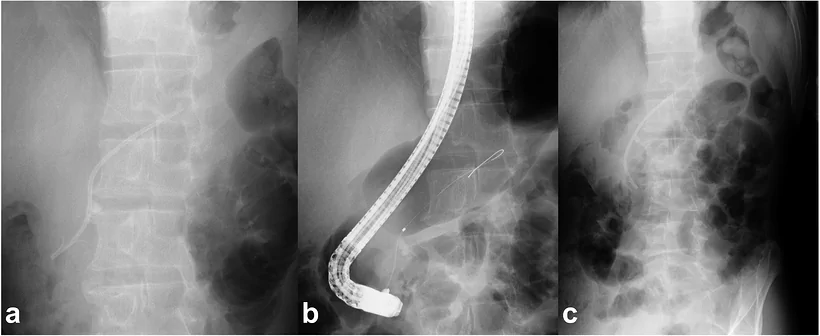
Radiographic and fluoroscopic findings during exchange of a pancreatic duct stent retained for 13 years. (a) Radiographic imaging showing the long‐term indwelling pancreatic duct stent that had been retained for 13 years. A pancreatic stone is shown adjacent to the retained stent. (b) A guidewire was inserted into the pancreatic duct, and endoscopic removal of the retained pancreatic duct stent was performed. No firm adhesion between the stent and the pancreatic stone was observed, and there was no evidence of stent fragmentation due to material deterioration during removal. (c) After removal of the retained stent, a new 7‐Fr 10‐cm pancreatic plastic stent was placed across the pancreatic duct stricture. Although stent insertion was technically challenging because of the pancreatic stone and ductal narrowing, successful stent placement was achieved.
